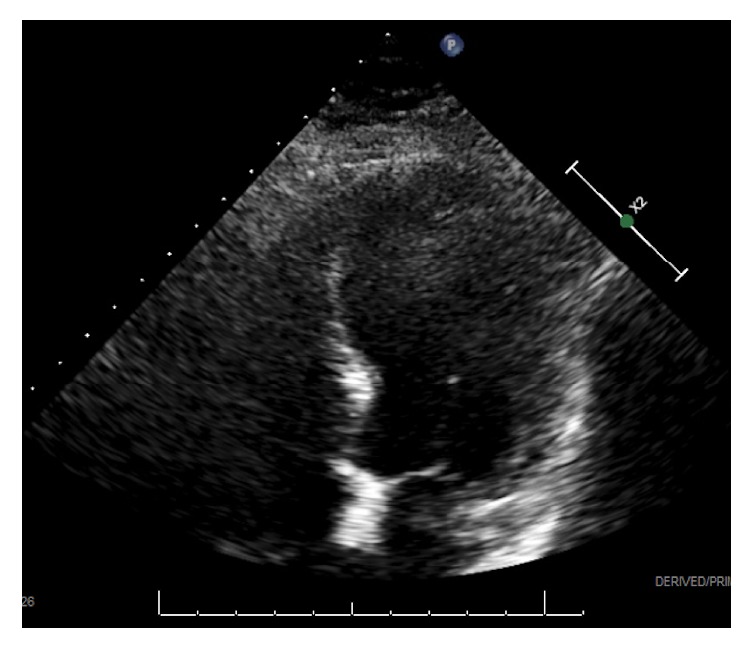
Apical four-chamber view demonstrating mid to apical ballooning of left ventricle.
